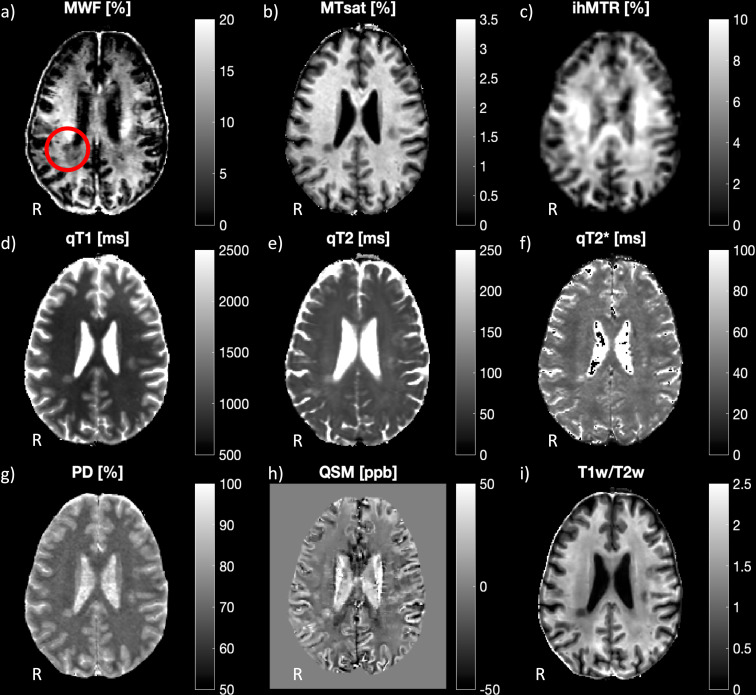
Representative parameter maps for all nine MR biomarkers in an MS patient. a) myelin water fraction (MWF), b) magnetization transfer saturation (MTsat), c) inhomogeneous MT ratio (ihMTR), d) qT1, e) qT2, f) qT2 * , g) proton density (PD), h) quantitative susceptibility mapping (QSM), and i) ratio between T1-weighted and T2-weighted images (T1w/T2w). A representative periventricular lesion is indicated by a red circle in the MWF map. Orientation is indicated with R for right.
